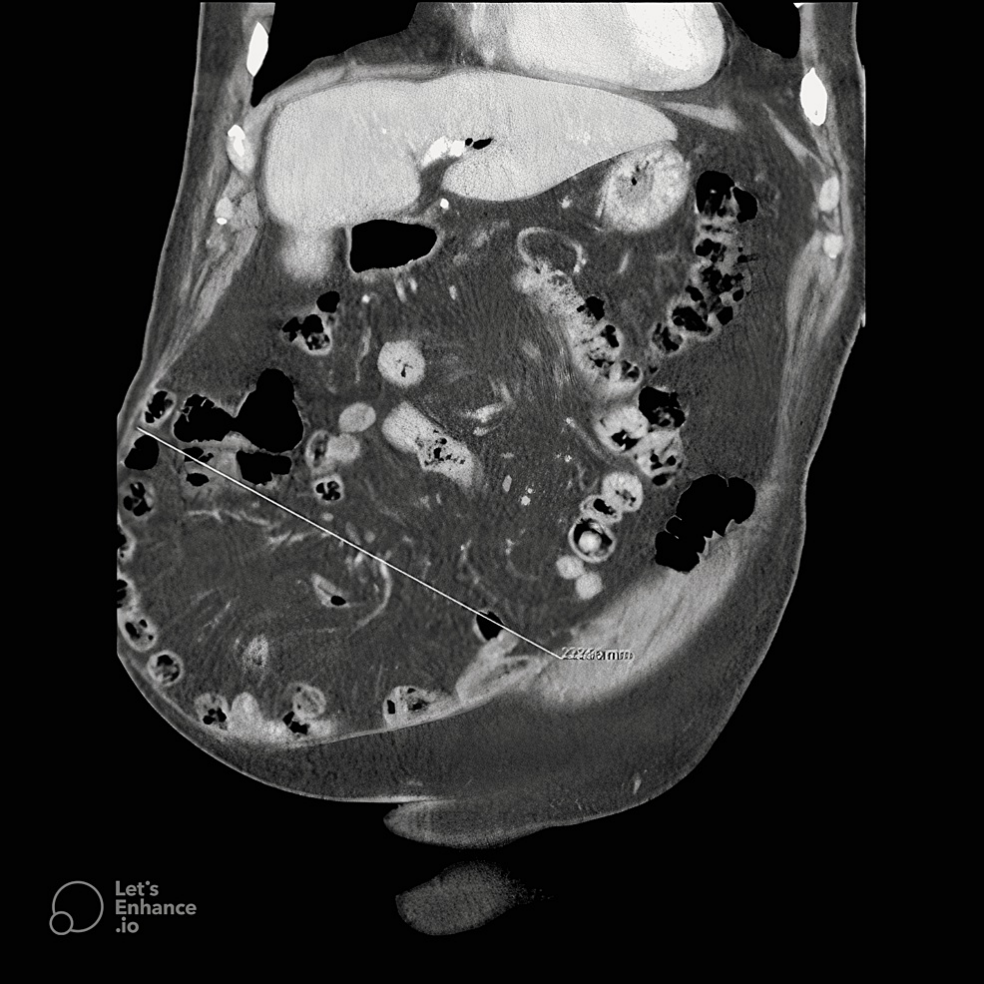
Coronal section CT image of the patient with approximately 24 cm fascial defect
